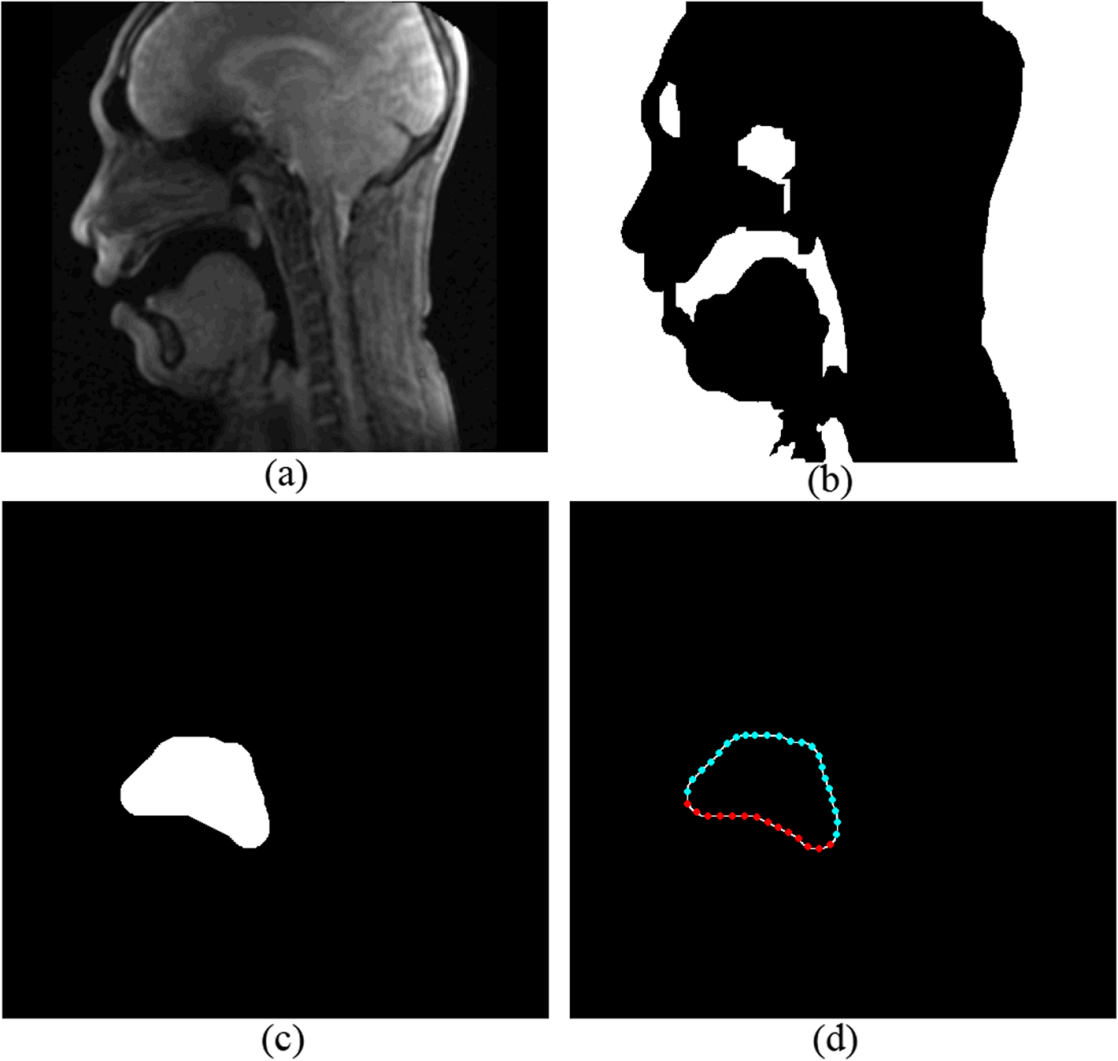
(a) One of the MRI frames of male subject investigated in this study; (b) the cavities obtained after the preprocessing operations; (c) a shape obtained from the oral cavity to provide the initial points of the active contour; and (d) the initial points of the active contour (lower in red and upper in yellow).
